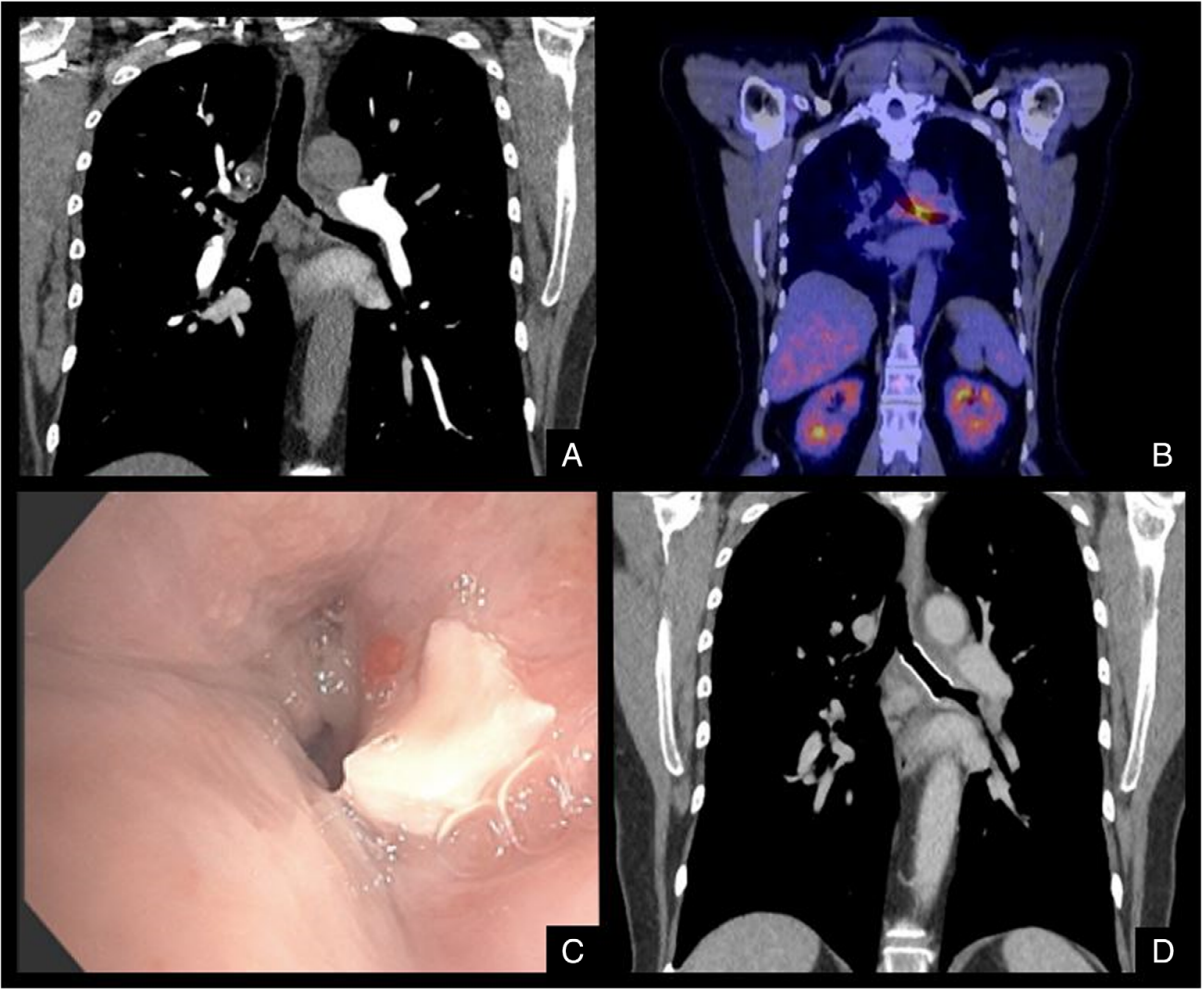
(A) Mediastinal view of computed tomography (CT) pulmonary angiogram showing mass in the left main bronchus (LMB). (B) Positron emission tomography/CT scan showing fluorodeoxyglucose uptake at the LMB and subcarinal, mediastinal and hilar lymph nodes. (C) Bronchoscopic image identifying obstructed LMB from the carina. (D) Coronal CT chest showing a metallic stent in the LMB
